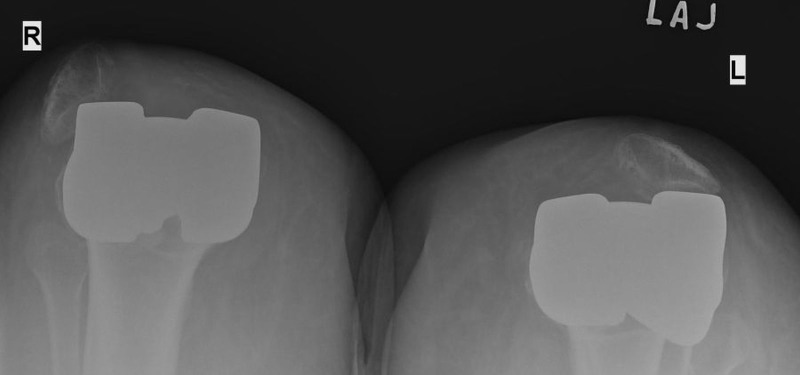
Skyline x-ray view of both knees showing the bilateral dislocation of the patellas.
